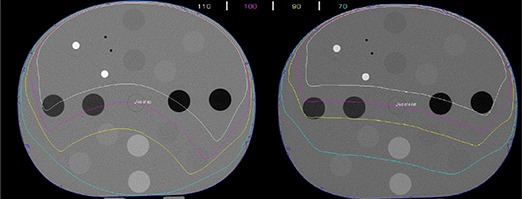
The relative dose distribution for the TBL (left) and IBL (right) CBCT. The 100% line intersects the isocenter.
